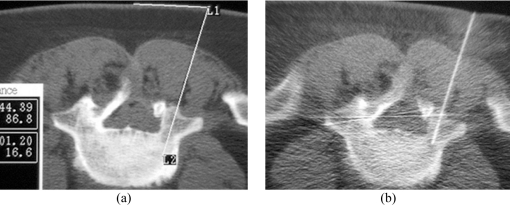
Planning of the approach and the needle route. (a) Preliminary axial CT image shows measurement of the distance between the proposed puncture site and the midline, as well as the depth and the angulation to targeted biopsy site in L5 vertebral body. (b) Axial CT fluoroscopic image taken with the patient lying prone shows the Ostycut needle inserted into the same vertebral body via the right transpedicular route.
